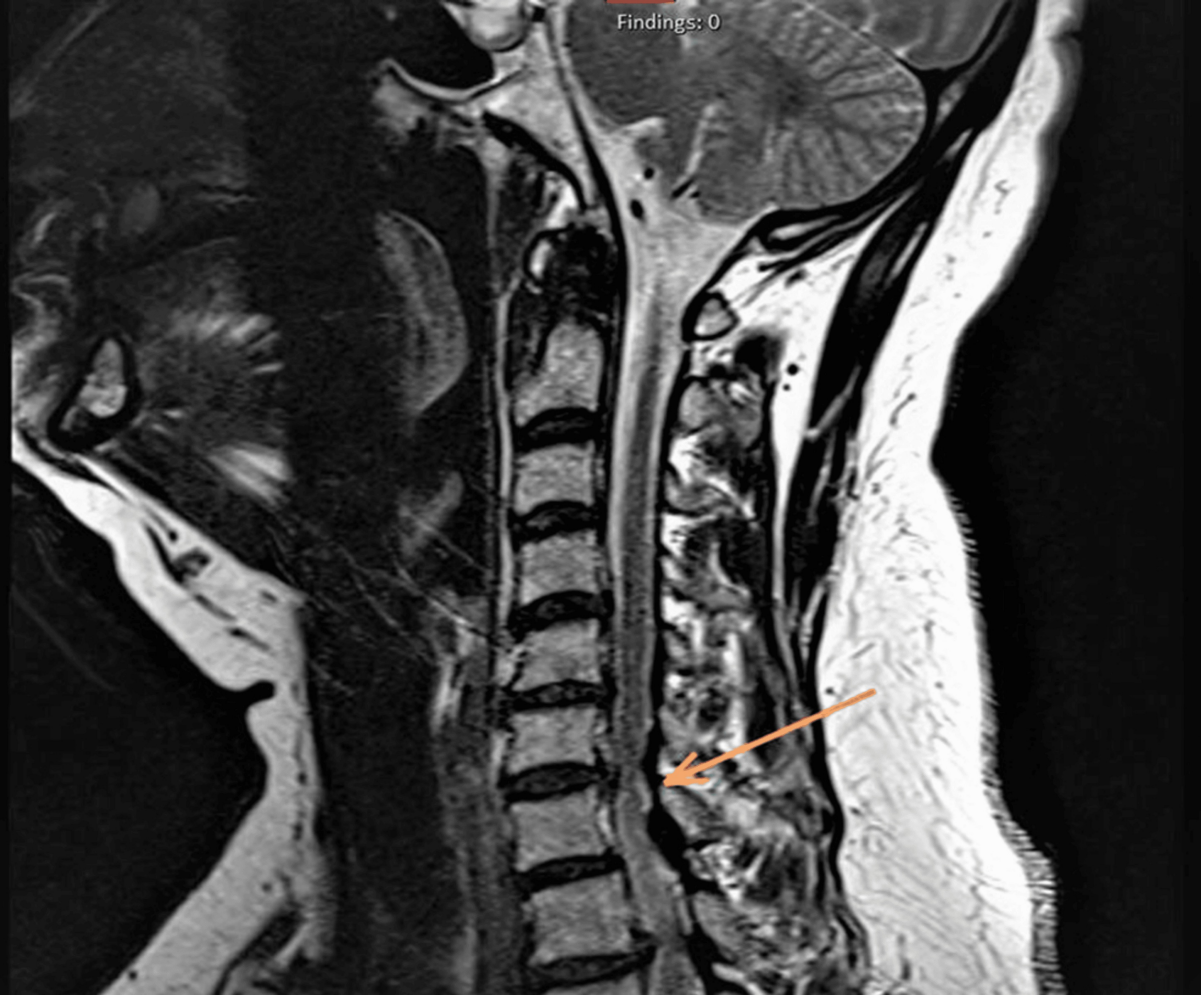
Representative image of MRI cervical spine showing severe foraminal stenosis at C6–C7 Image Source: Wasey et al., 2022 [11]; under the Creative Commons Attribution 3.0 Unported licence (https://creativecommons.org/licenses/by/3.0/)
